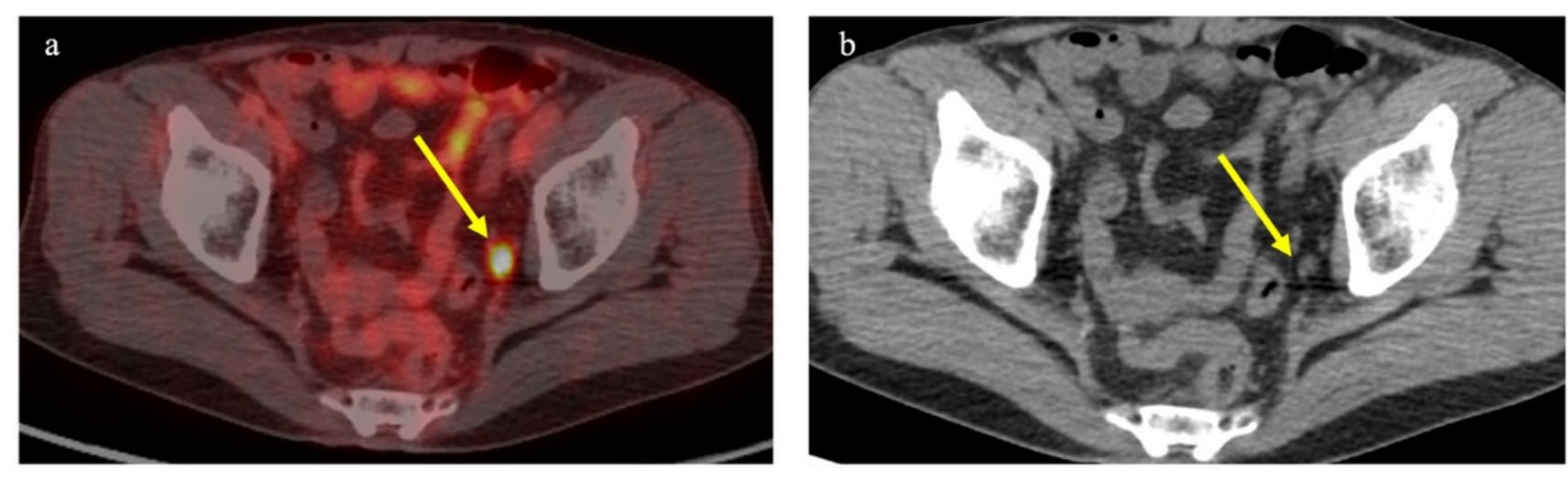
PET/CT using ^18^F-FSU-880 in a 71-year-old man with biochemical recurrence after total prostatectomy (pT2bN0, Gleason score 4 + 3). PSA level increased to 2.10 ng/ml. Focal uptake was observed in a left obturator lymph node (SUVmax; 10.2). Salvage radiation therapy had been considered before scanning, but this treatment plan was subsequently adjusted to hormonal therapy. a Transaxial PET/CT fusion image. b Transaxial CT
